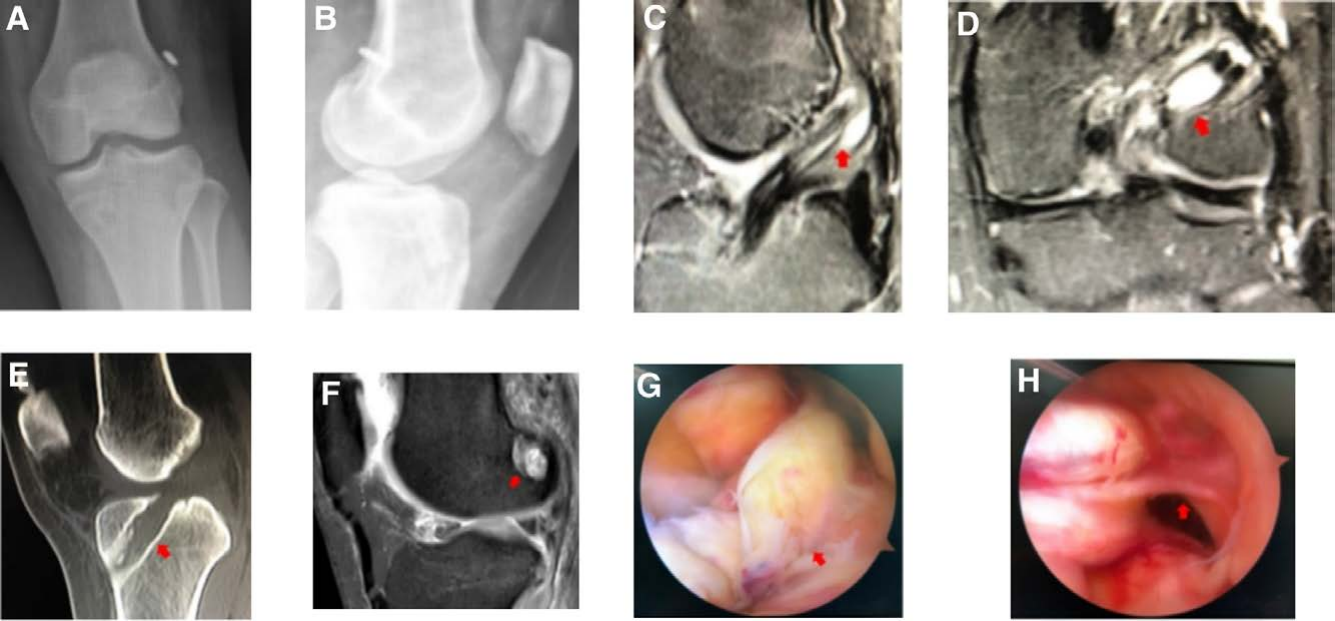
(A and B) DR findings: The EndoButton plate was not tightly positioned close to the periosteum, and the femoral and tibial tunnels were in good positions. (C and D) MRI findings 6 months after the operation: the femoral tunnel portal and tendon showed relatively high signal intensity. In particular, the proportion of high signal intensity in the femoral tunnel exceeded 50%, indicating ligamentization failure in the tendon. (E) CT showed that the tibial and femoral tunnels were significantly enlarged, with locally hardened edges. (F) MRI after the second injury revealed a tear in the posterior horn of the medial meniscus. (G and H) Intraoperative exploration revealed the presence of a fatty ACL and tendon vacancy in the femoral tunnel. Additionally, the shape and tension of the lateral meniscus were deemed satisfactory. The RAM region of the medial meniscus exhibited a tear resembling a barrel handle, which was subsequently repaired and secured with sutures. ACL = anterior cruciate ligament.
